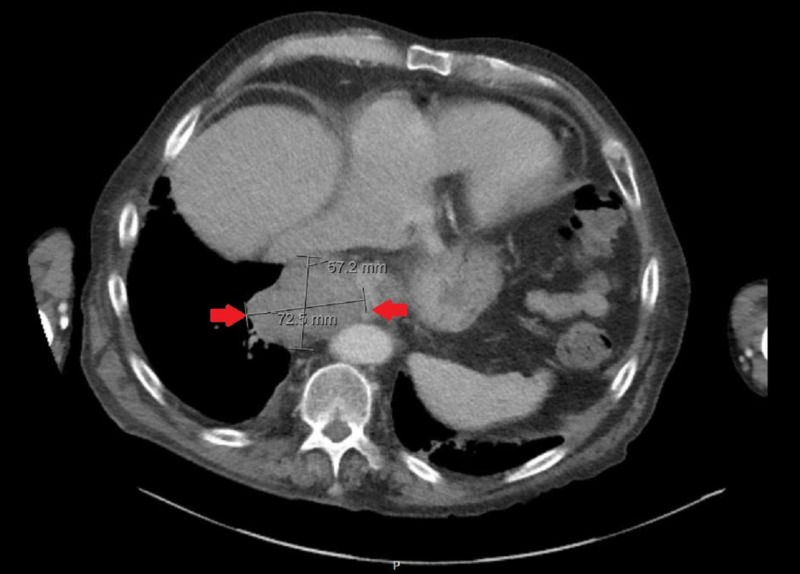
CT thorax with contrast showing 7.5-cm mass at the GE Junction (between red arrows). GE: Gastroesophageal
